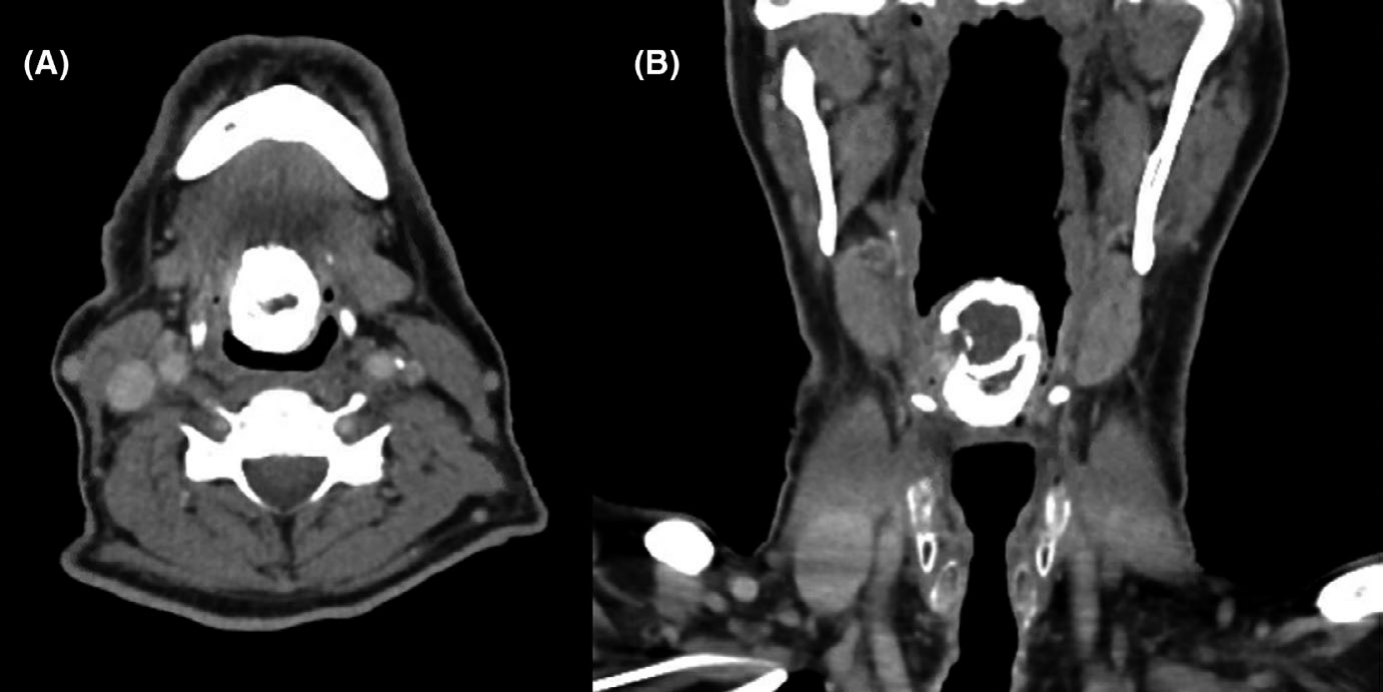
CT neck soft tissues with IV contrast: A, Axial view of rim‐calcified mass at midline of tongue base measuring 30 × 32 mm in anteroposterior diameter; B, Coronal view of rim‐calcified mass at tongue base measuring 40 mm in lengt
